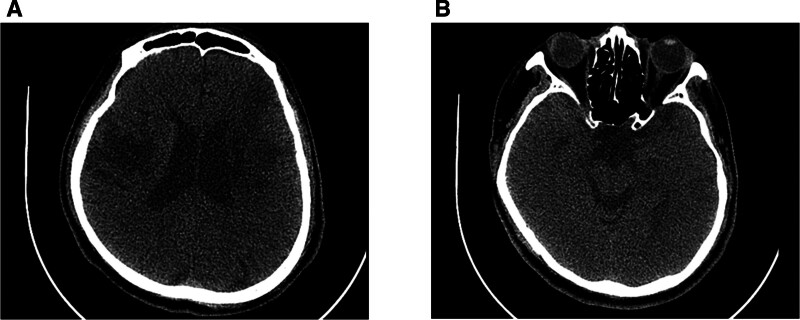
Brain CT images obtained 8 months after the second cerebral infarction. (A) Chronic infarct lesions are observed in both cerebral hemispheres; (B) No definite abnormal changes are noted in the brainstem region. CT = computed tomography.
